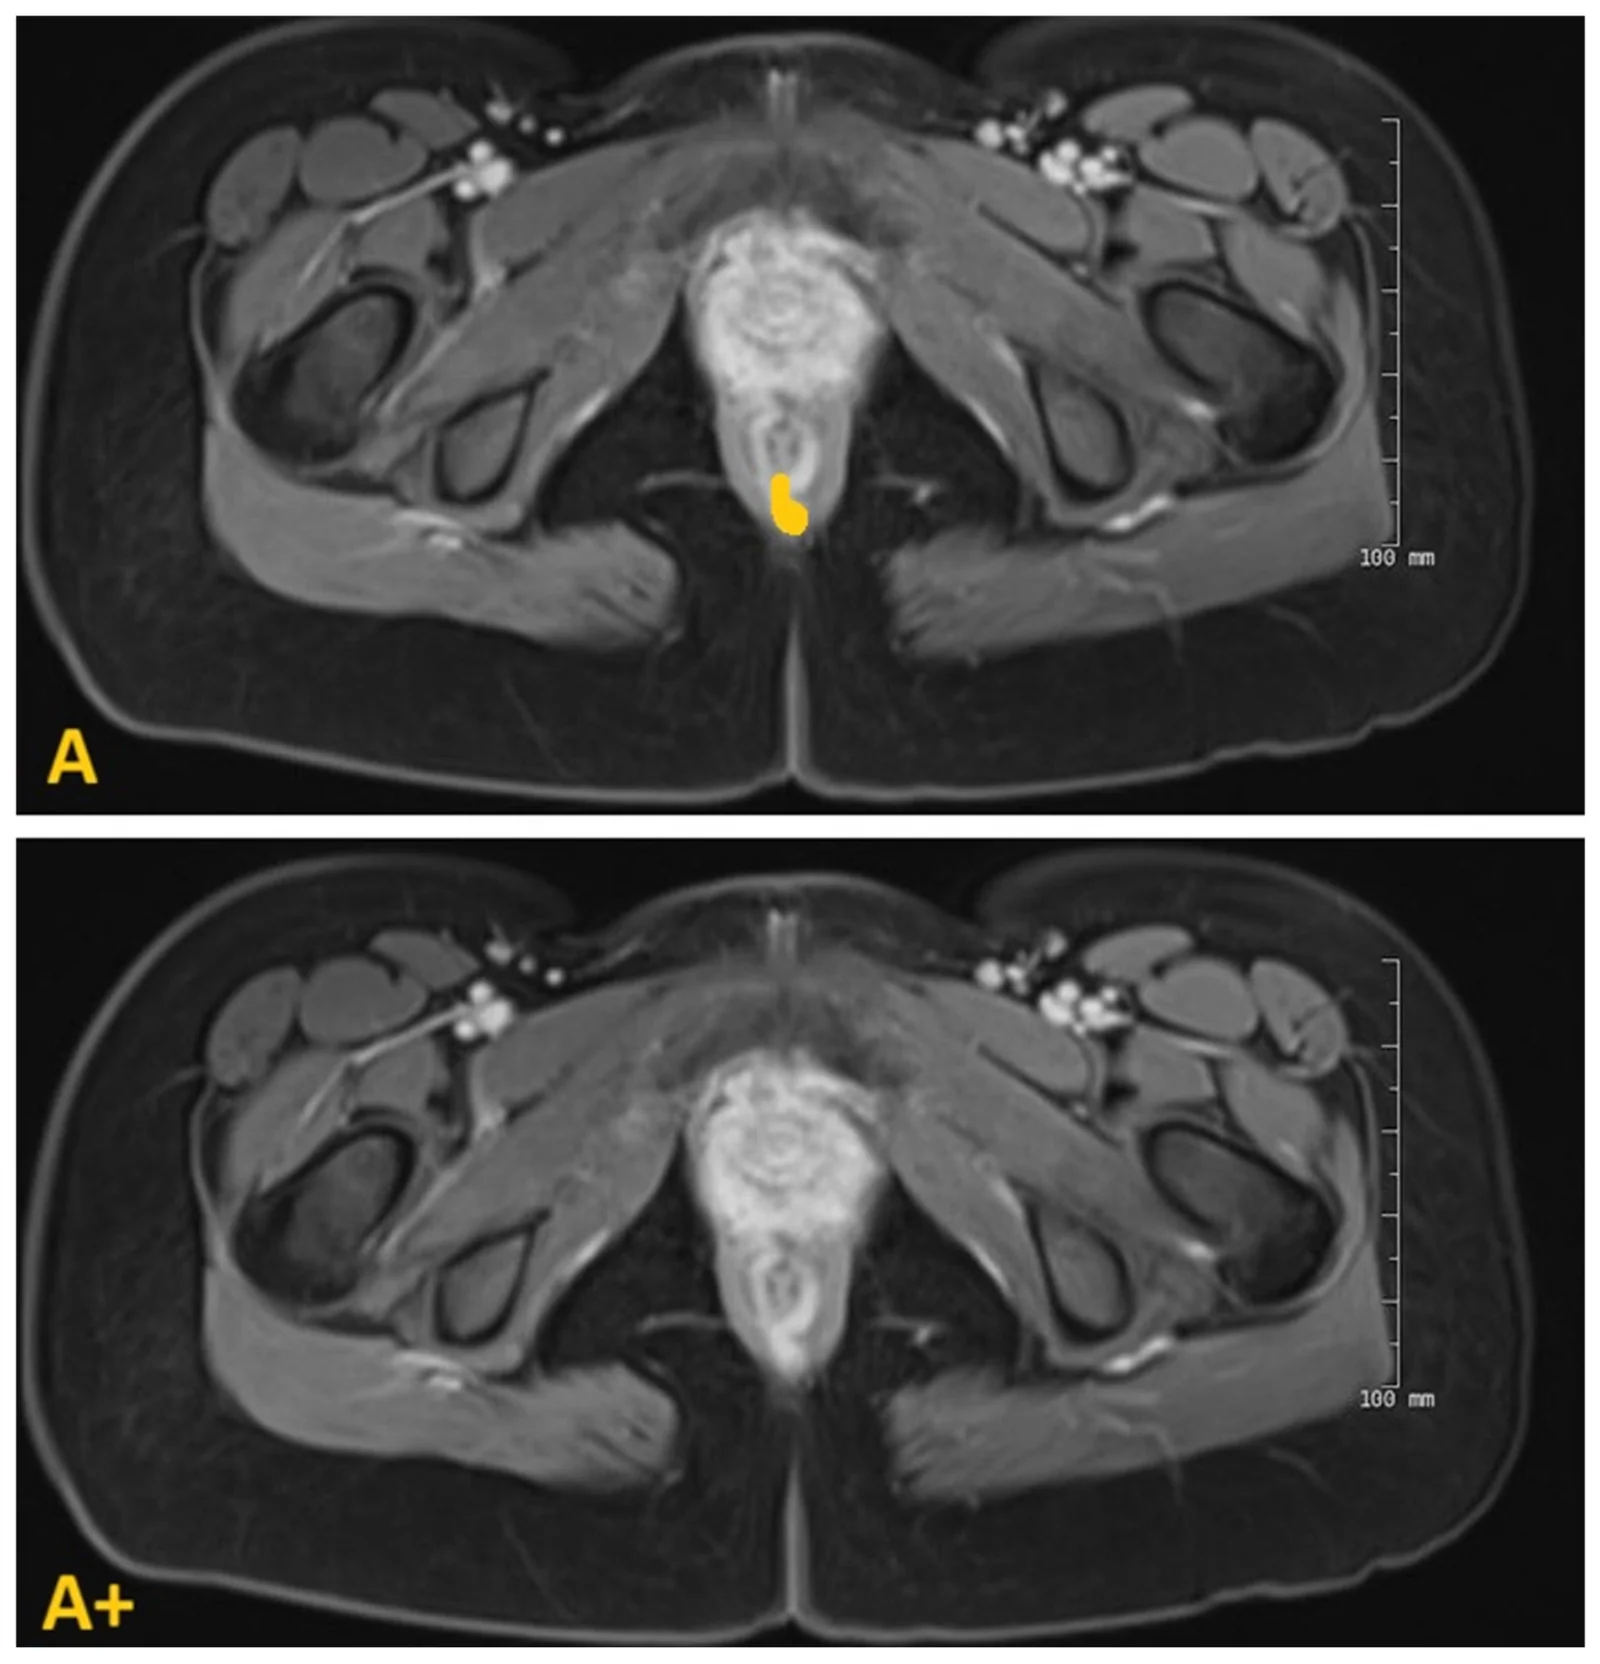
Selected magnetic resonance images of another patient with Crohn’s disease shown in Figure 26. (A) Axial post-contrast T1-weighted image showing a small blind-ending sinus tract (filled with yellow color) arising from the posterior midline around the anal canal. (A+) Corresponding unannotated image.
